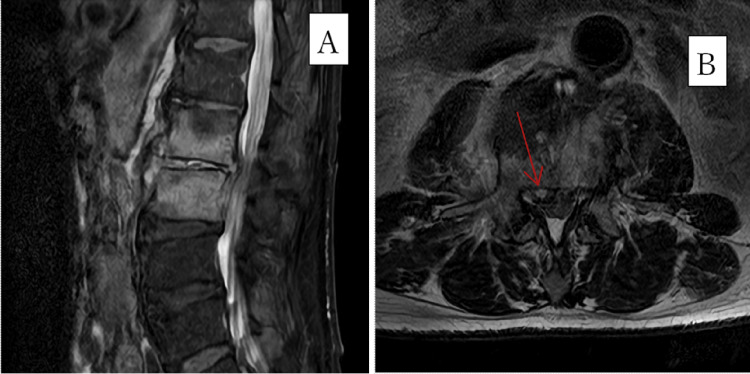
Magnetic resonance imaging findings of lumbar spondylodiscitis and epidural abscess (A) Sagittal magnetic resonance imaging of the lumbar spine demonstrating abnormal signal intensity involving the L2-L3 intervertebral disc and adjacent vertebral endplates, consistent with spondylodiscitis. Endplate destruction and inflammatory changes are evident. (B) Axial contrast-enhanced magnetic resonance imaging demonstrating an epidural fluid collection (arrow) at the L3 level, compatible with an epidural abscess.
